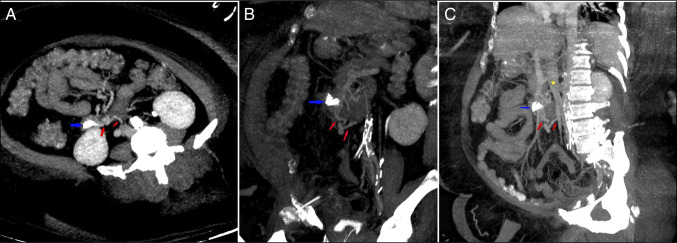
(A and B) Axial and coronal CT demonstrating endoscopic clips in the wall of the duodenum (blue arrow) with varix complex and draining outflow vein (red arrows). (C) Maximum intensity oblique coronal CT image demonstrating endoscopic clips in the wall of the duodenum (blue arrows) with varix complex and draining outflow vein (red arrows). Inferior vena cava (yellow star). CT, computed tomography.
